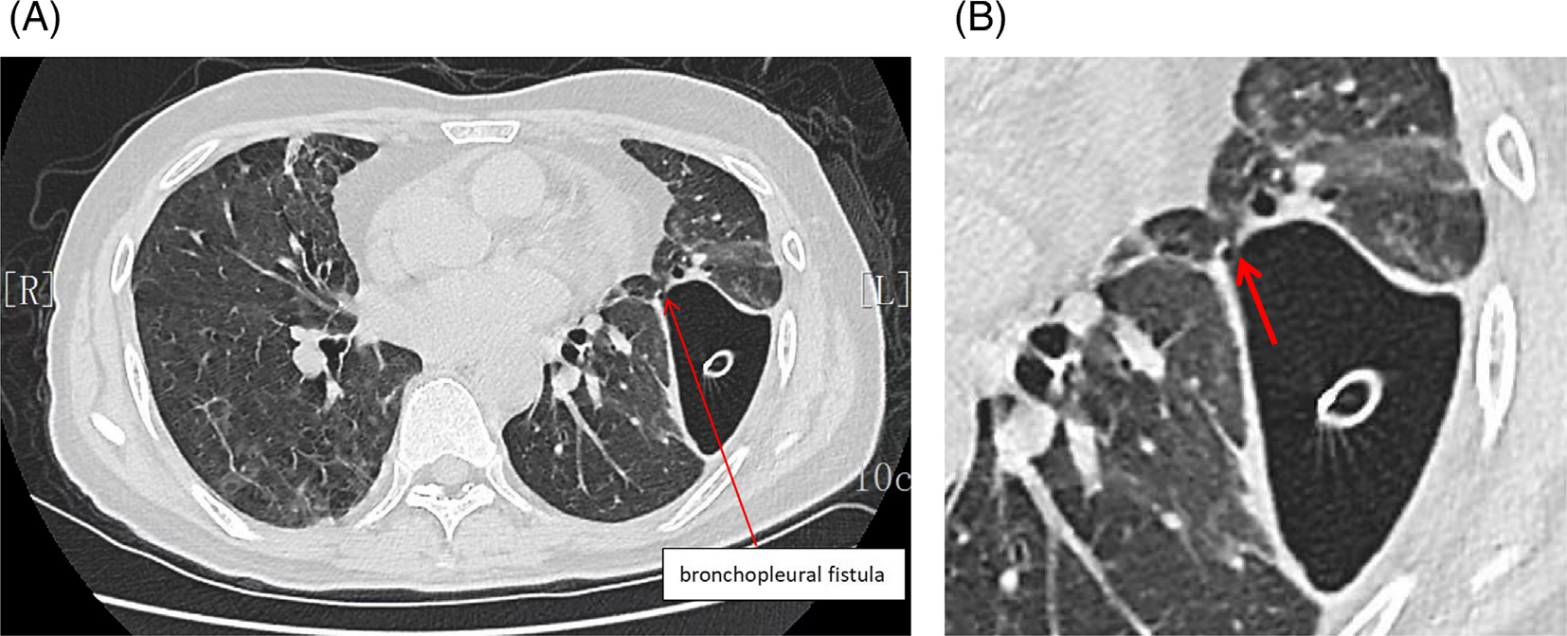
(A) There was a 1.41 mm diameter fistula in the left superior lobe of the lung connecting to the chest cavity. (B) Enlarged chest CT image of a bronchopleural fistula.
